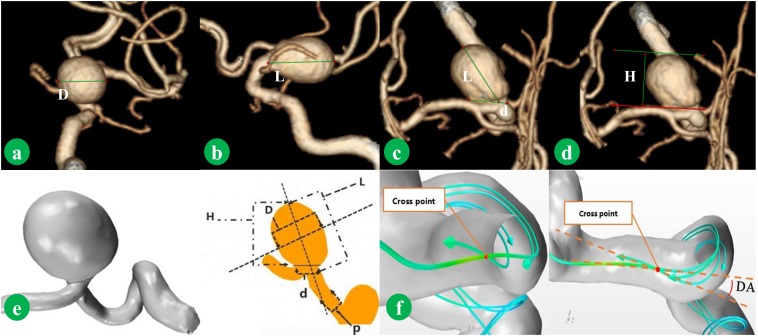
Morphological parameters were measured from CTA image. D is the maximum diameter of the body (a). L is the maximum distance of the dome from the neck plane (b). d is the average diameter of the neck (c). H is the maximum perpendicular distance of the dome from the neck plane (d). The method of measurement is shown in (e). p is average diameter of the parent artery. Normal vector was combined. The deviated angle (DA), which was between co-velocity and normal vector, was measured (f).
